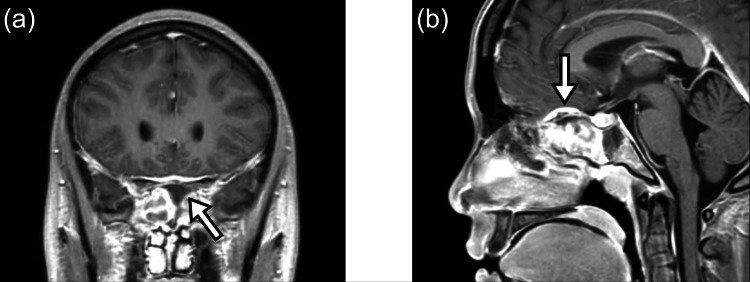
Contrast-enhanced magnetic resonance imaging (MRI) on day 5 (a) Marked mucosal thickening, enhancement, and fluid retention in the left sphenoid sinus (arrow); (b) Localized dural thickening near the anterior cranial base (arrow), suggesting inflammatory extension.
